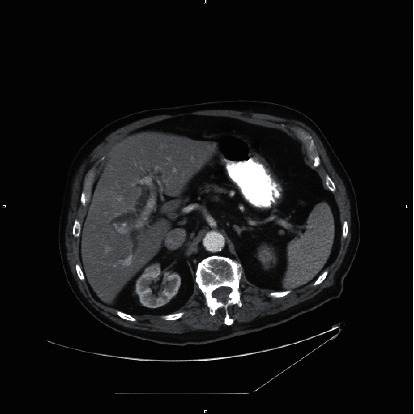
Axial CT of the abdomen with contrast demonstrates intrahepatic biliary dilatation.
